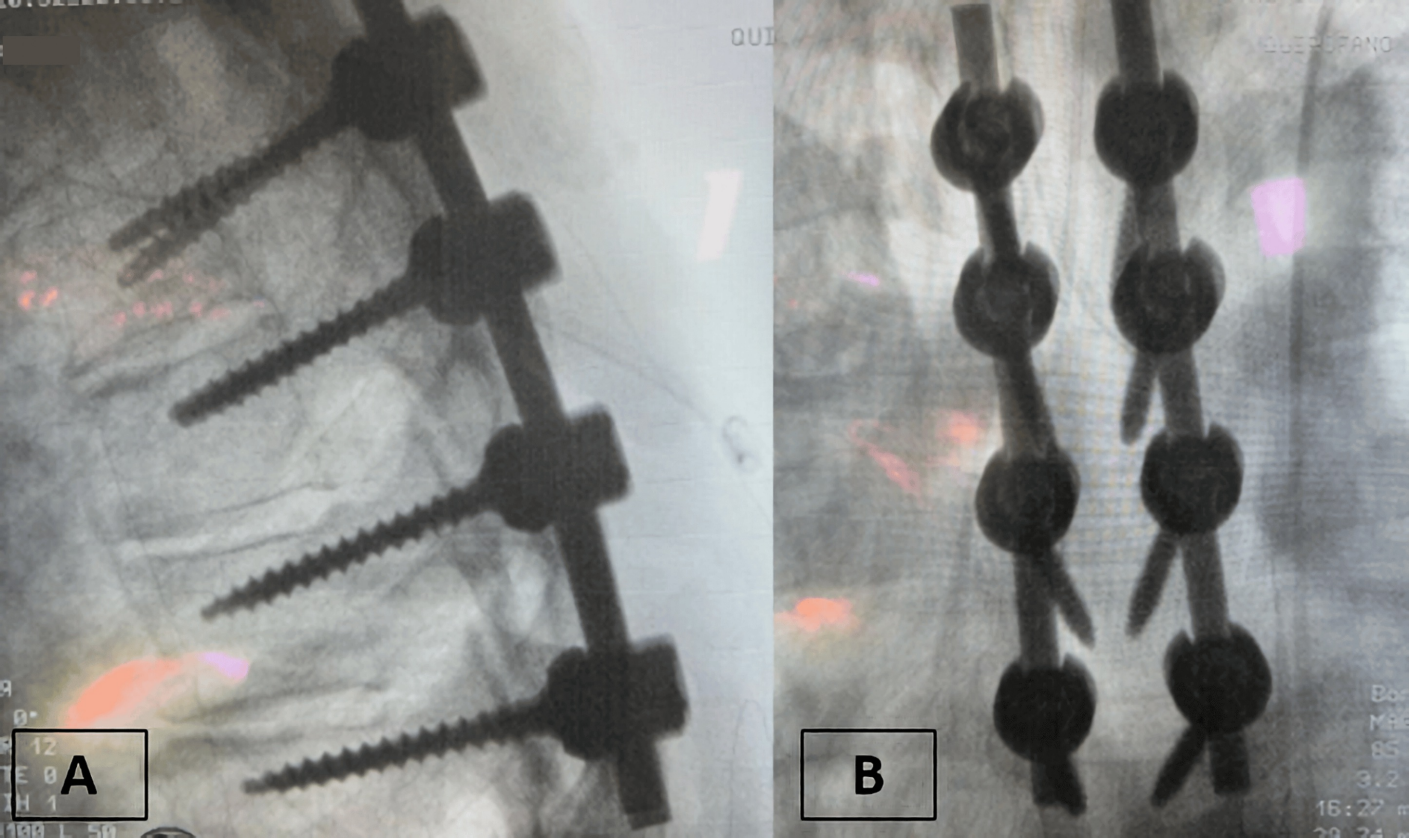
Postoperative radiographs of thoracic spine instrumentation. (A) Lateral view showing correct placement of transpedicular screws and titanium rods providing stabilization of the thoracic spine. (B) Anteroposterior view confirming symmetrical alignment of transpedicular screws and rods across the instrumented levels.
